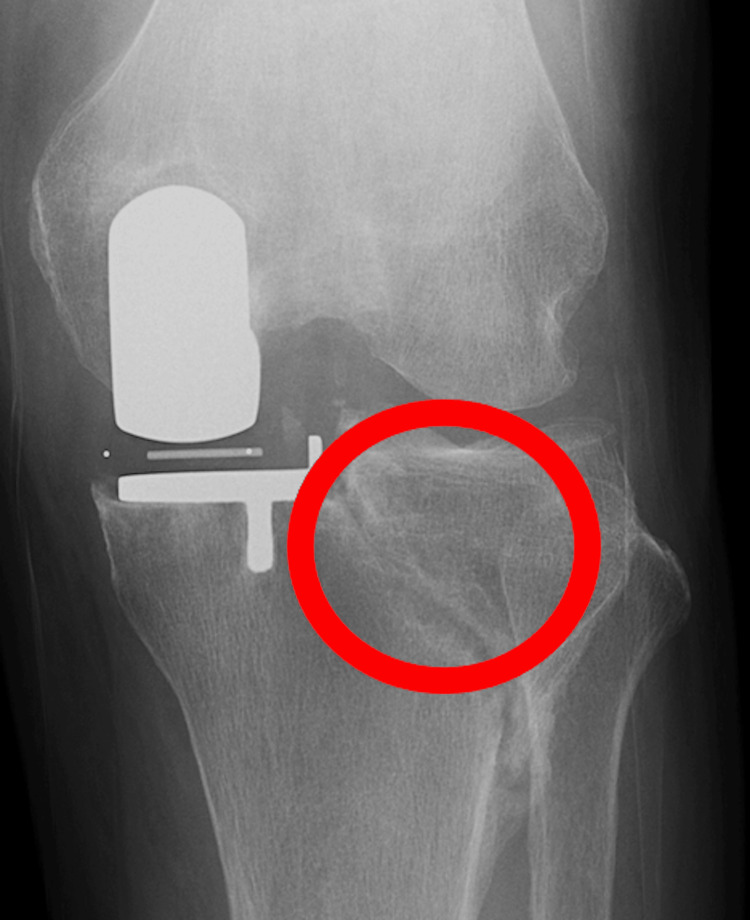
Complete bone union with no displacement is shown on radiographs 6 months postoperatively (circle).
